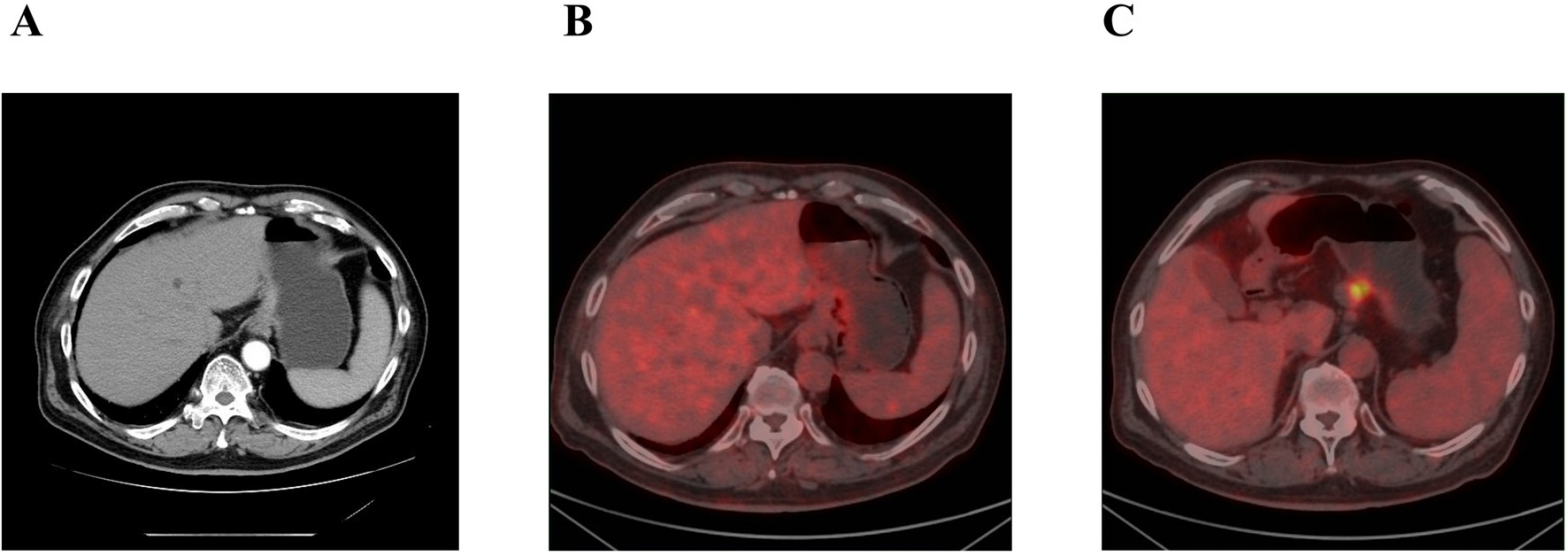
(A) Abdominal enhanved CT: uneven thickening augmentation of the gastric fundus. (B, C) PET-CT: (B) thickening of the gastric wall. (C) lymph node with increased metabolism of FDG.
